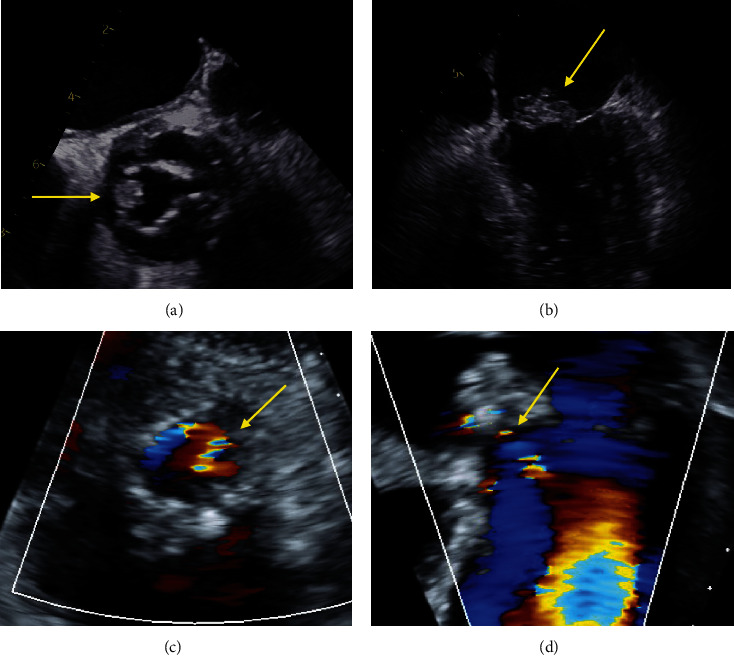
Select images from the (a, b) initial transesophageal echocardiogram performed upon admission to the referring hospital and (c, d) follow-up transthoracic echocardiogram performed upon transfer to our tertiary academic medical center. (a) Midesophageal short-axis view demonstrating thickened bioprosthetic aortic valve leaflets with vegetation (arrow). (b) Midesophageal four-chamber view showing large vegetation along the anterior leaflet of the mitral valve. (c) Parasternal short-axis view of bioprosthetic aortic valve in diastole with color flow suggestive of aortic valve regurgitation (arrow). (d) Apical five-chamber view in diastole showing high-velocity flow in the left ventricular outflow tract consistent with aortic valve regurgitation (arrow).
